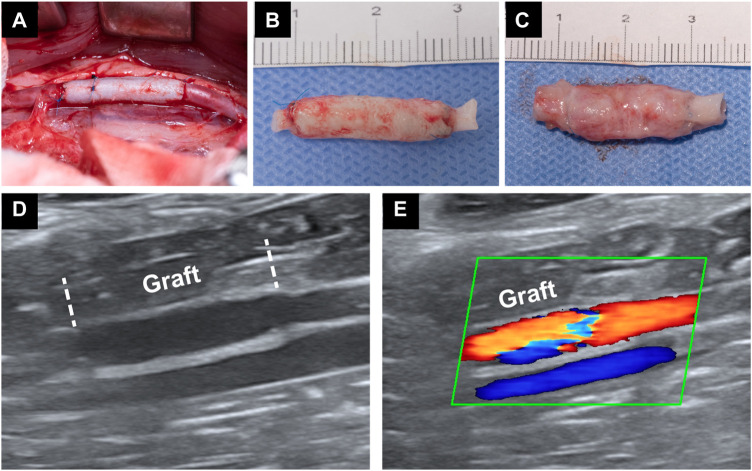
Rabbit abdominal aorta interposition model. (A) is a photo of the implanted graft immediately after the surgery. (B) is a photo of a graft which was implanted for 1 week. (C) is a photo of a graft which was implanted for 3 months. (D,E) are the Doppler ultrasound images of an implanted graft.
